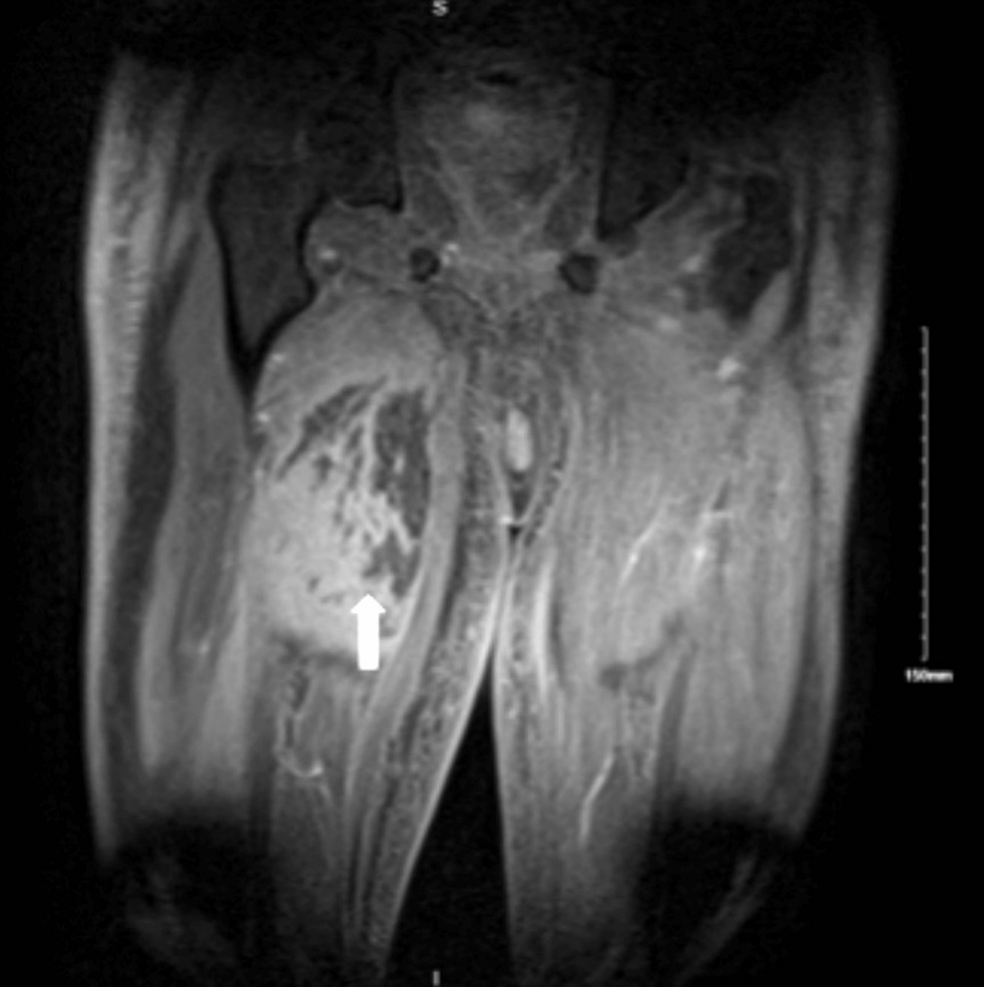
MRI of the right thigh in coronal view with contrast showing severe myositis with associated superficial and deep fasciitis and the areas of myonecrosis MRI: magnetic resonance imaging
